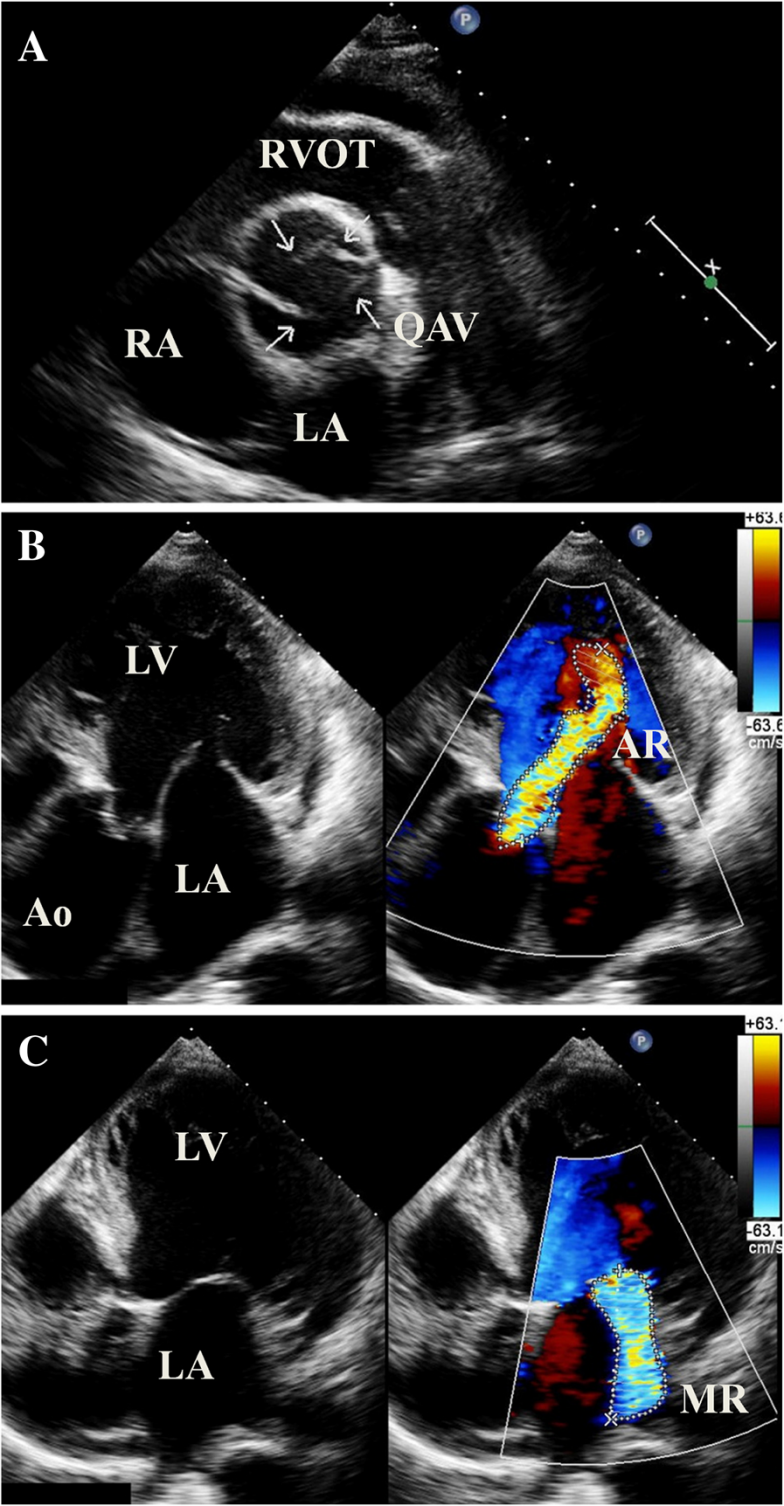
Echocardiography of a 58-year old man with quadricuspid aortic valve (QAV). Figure 1A, short axis view shows the four cusps of aortic valve; Figure 1B, severe aortic regurgitation and dilated aortic root; Figure 1C, mitral regurgitation. RVOT, right ventricular outflow tract; RA, right atrium; LA, left atrium; LV, left ventricle; Ao, Aorta; AR, aortic regurgitation; MR, mitral regurgitation.
